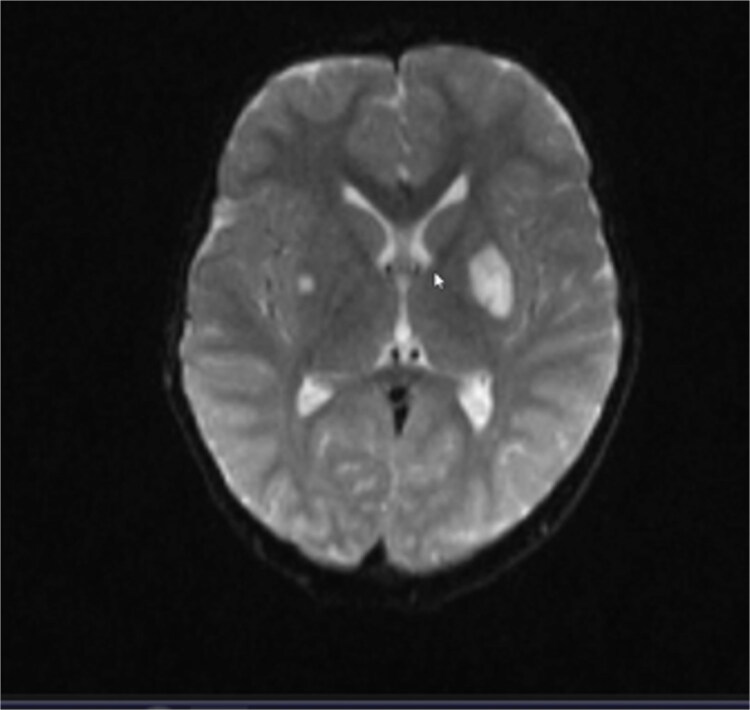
Brain MRI unveiled the presence of diffuse periventricular white matter lesions.
